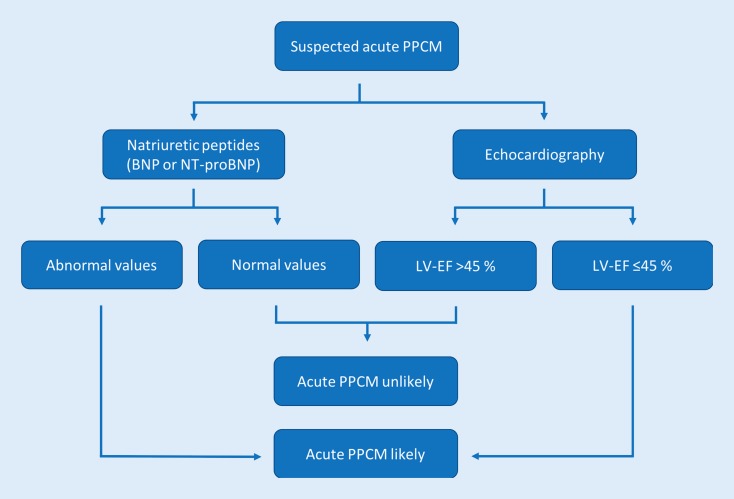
Pragmatic evaluation scheme for suspected acute PPCM during the end of pregnancy or the months after delivery. Measurement of natriuretic peptides and transthoracic echocardiography are recommended to quickly strengthen or rule out the diagnosis of PPCM. *PPCM* peripartum cardiomyopathy, *LVEF* left ventricular ejection fraction, *BNP* brain natriuretic peptide, *NT-proBNP* N-terminal prohormone brain natriuretic peptide
